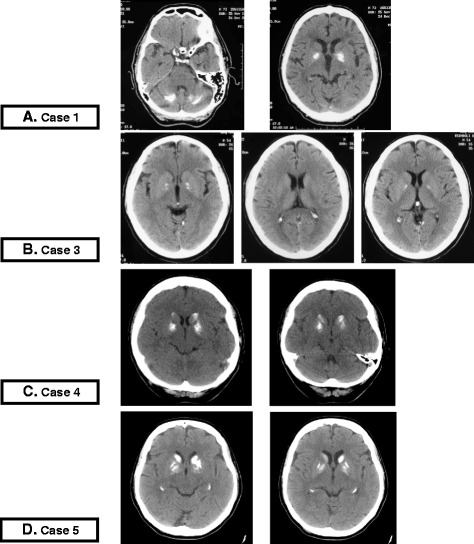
CT scan imaging of Cases 1,3,4 and 5. a Case 1. CT scans: extensive bilateral calcification of pallidus nuclei of basal ganglia and dentate nuclei of cerebellum; Hypodense malacic aspect in left frontal lobe. b Case 3. CT scans: mineral hyperdense deposits in bilateral pallidus nuclei without other area of altered density. Small enlargement, age-related, of frontal cerebral sulci due to atrophy. c Case 4. CT scans: hyperdense simmetric calcific deposits in pallidus nuclei and caudate nuclei of both sides. d Case 5. CT scans: hyperdense bilateral calcific deposits in the head of caudate nuclei, putamen and pallidus nuclei
